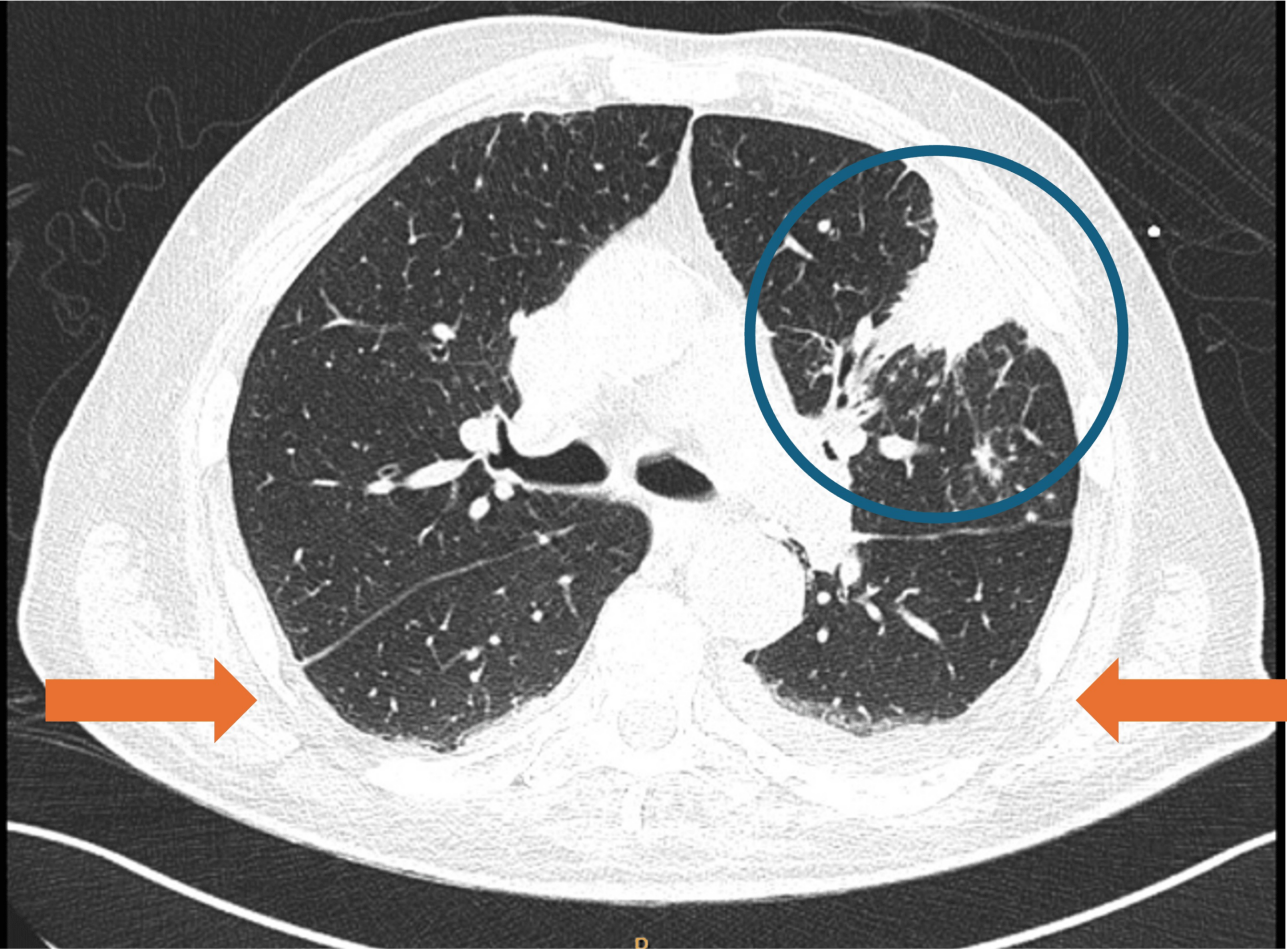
Axial chest CT demonstrating improvement of left upper-lobe consolidation with reduction of the cavitary component (blue circle) and decreased bilateral pleural effusions (orange arrows).
